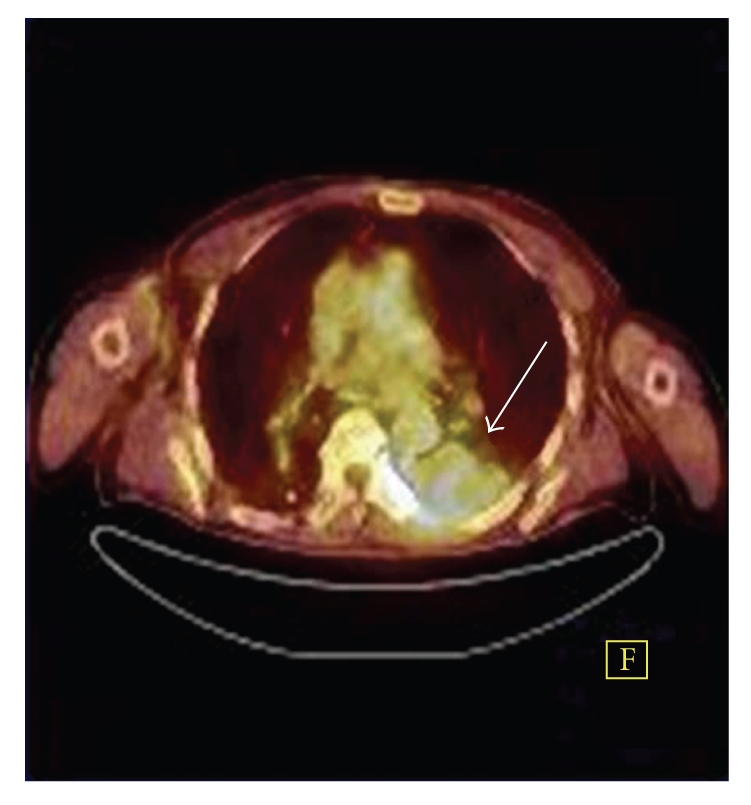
Axial-fused PET/CT image shows a pleural-based tumor with a calculated SUVmax of 4.36 (arrow).
